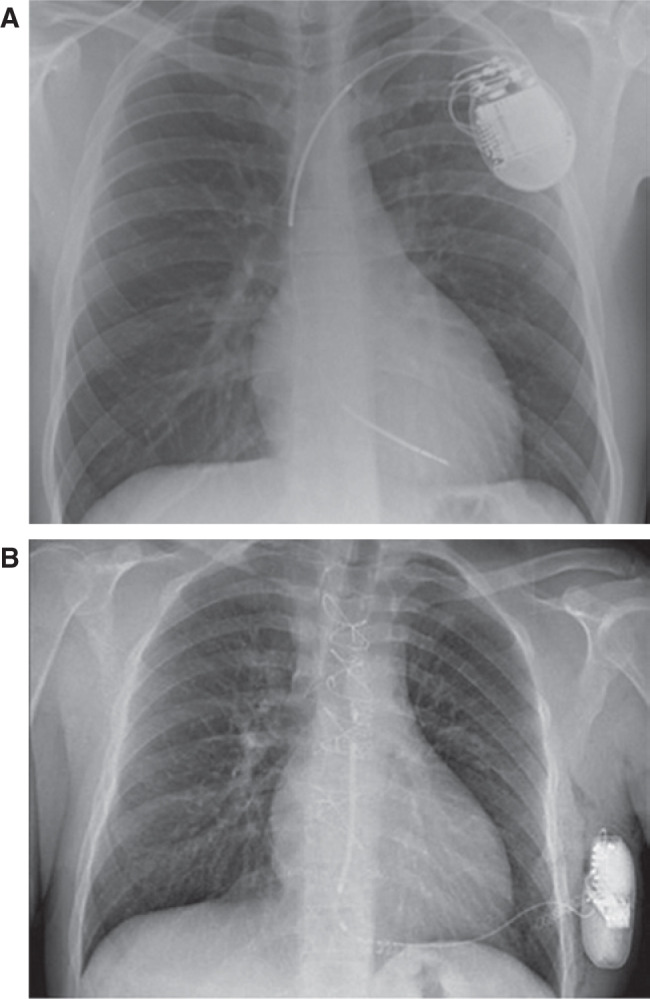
Chest radiographs demonstrating the differences between transvenous and subcutaneous ICD systems. A: Transvenous ICD. B: S-ICD.
